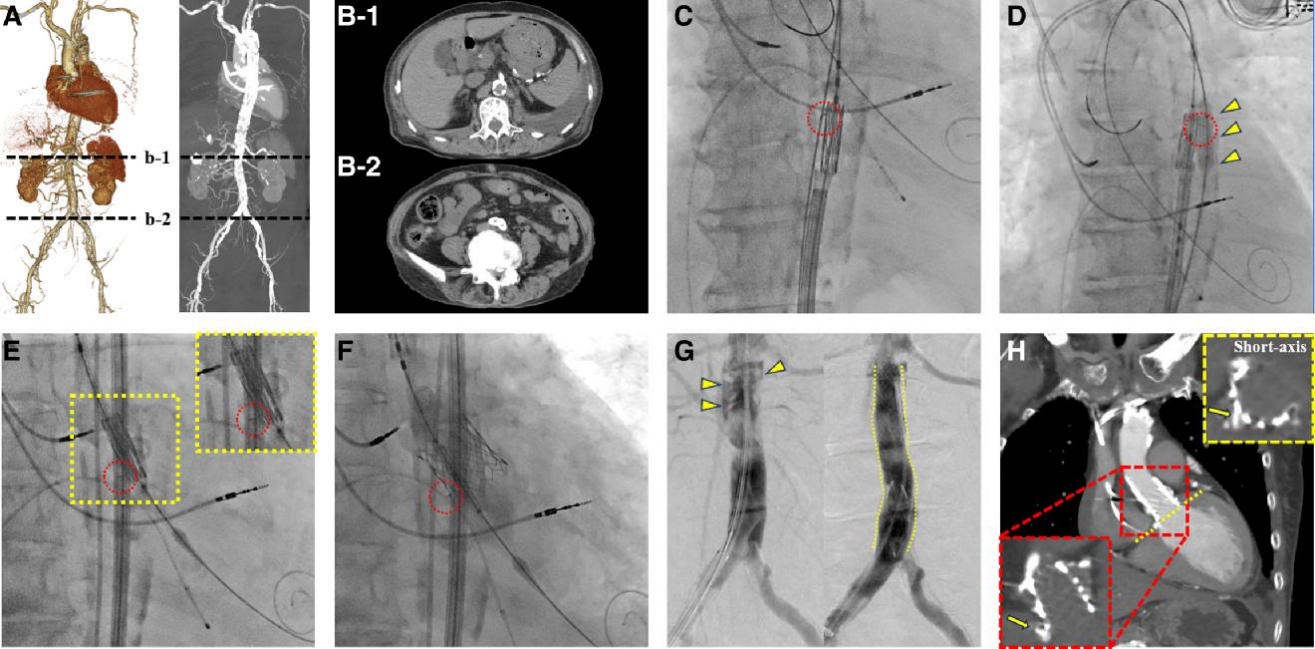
(*A*) Three-dimensional computed tomography (CT) angiography of transfemoral access. Abdominal aorta (*B*-1) and bilateral proximal iliac artery stenoses (*B*-2) with calcified plaque. (*C*) An outward bending of the stent frame (circle). (*D*) Balloon angioplasty (arrowheads) advanced via the contralateral femoral artery fails to fix the deformation (dotted circle). (*E*) Before and (*F*) after the deployment of the deformed valve (circle). (*G*) The angiogram after the valve deployment shows localized dissection in the abdominal aorta (arrowheads) for which endovascular stent grafts (dotted lines) are deployed for successful sealing. (*H*) CT scan after the procedure shows deformed valve (arrows) without any signs of aortic annular rupture.
